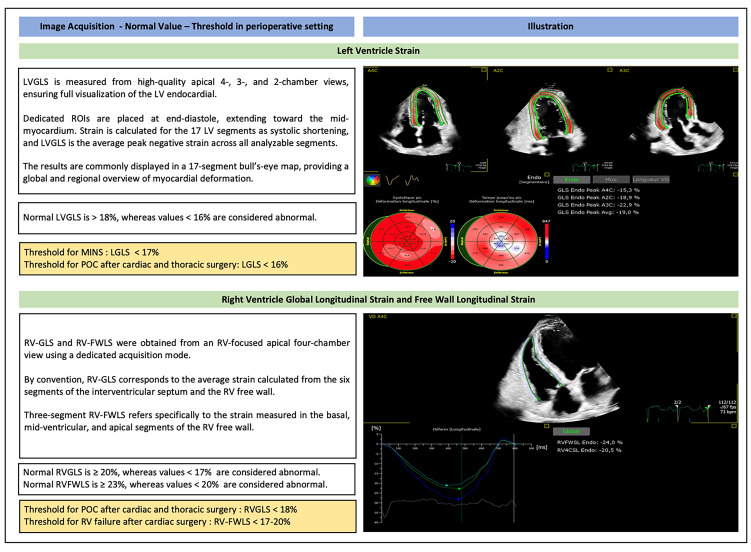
Ventricular strain assessment: acquisition, reference values, and risk thresholds. Left and right ventricular strain (LVGLS, RVGLS, RV free-wall strain), including acquisition views, normal ranges, and perioperative thresholds for MINS, RV failure, and hemodynamic instability. Representative strain curves and bull’s-eye maps are shown. For improved readability, strain values displayed in this figure have been normalized to absolute values, whereas strain values and thresholds throughout the manuscript are reported using their original negative polarity as generated by the ultrasound software.
